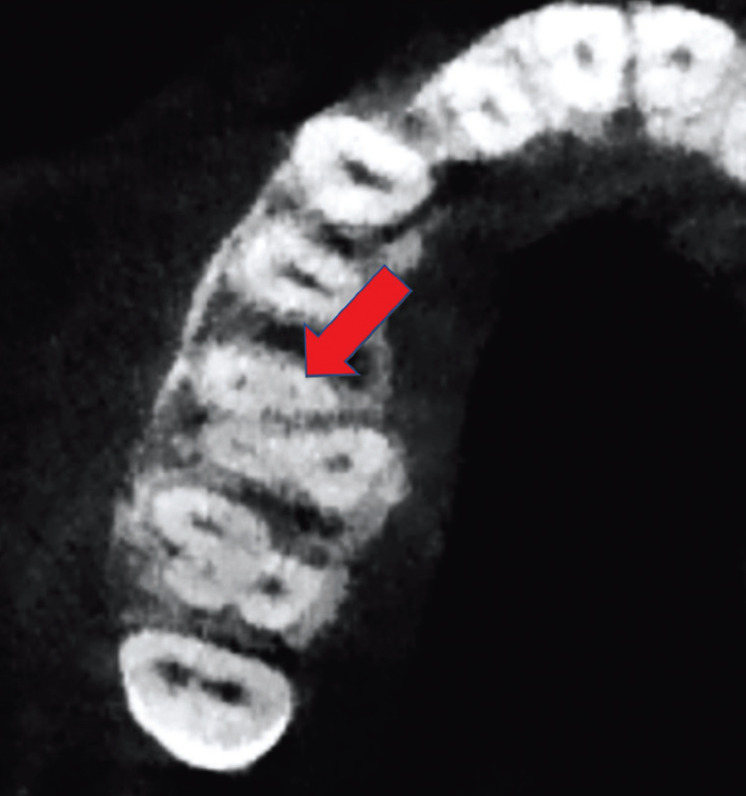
MB2 root canal location in a right maxillary first molar using CBCT.
